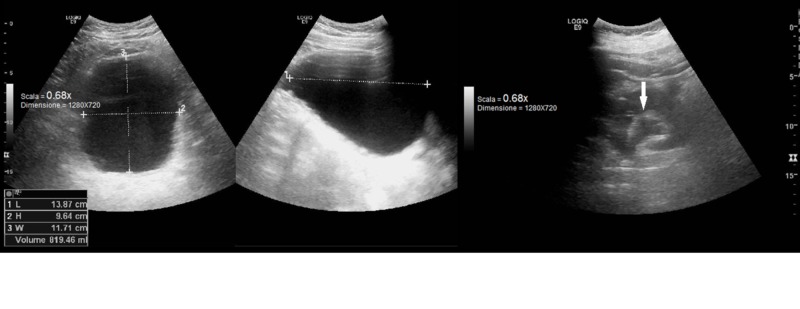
Abdomen ultrasound showing residual urine volume and first degree hydronephrosis (white arrow). L: Length; H: Height; W: Width.
